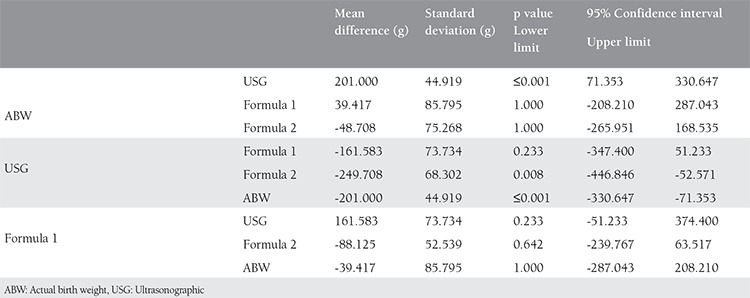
Comparison of actual birth weight (ABW), ultrasonographic, formula 1 and formula 2 byusing Bonferroni corrected multiple comparison test in patients with ABW ntsents
